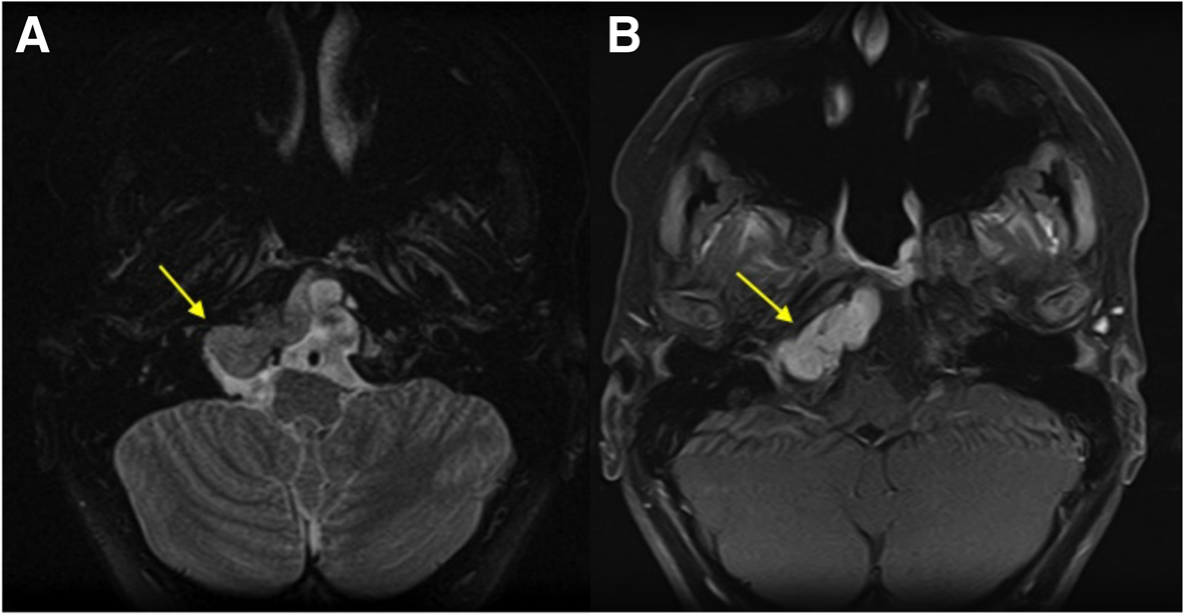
a Axial T2-weighted image of the recurrent tumor showing intermediate to dark, isointense to gray matter signal intensity (*arrow*). b Axial T1-weighted post-contrast image showing avid enhancement (*arrow*)
